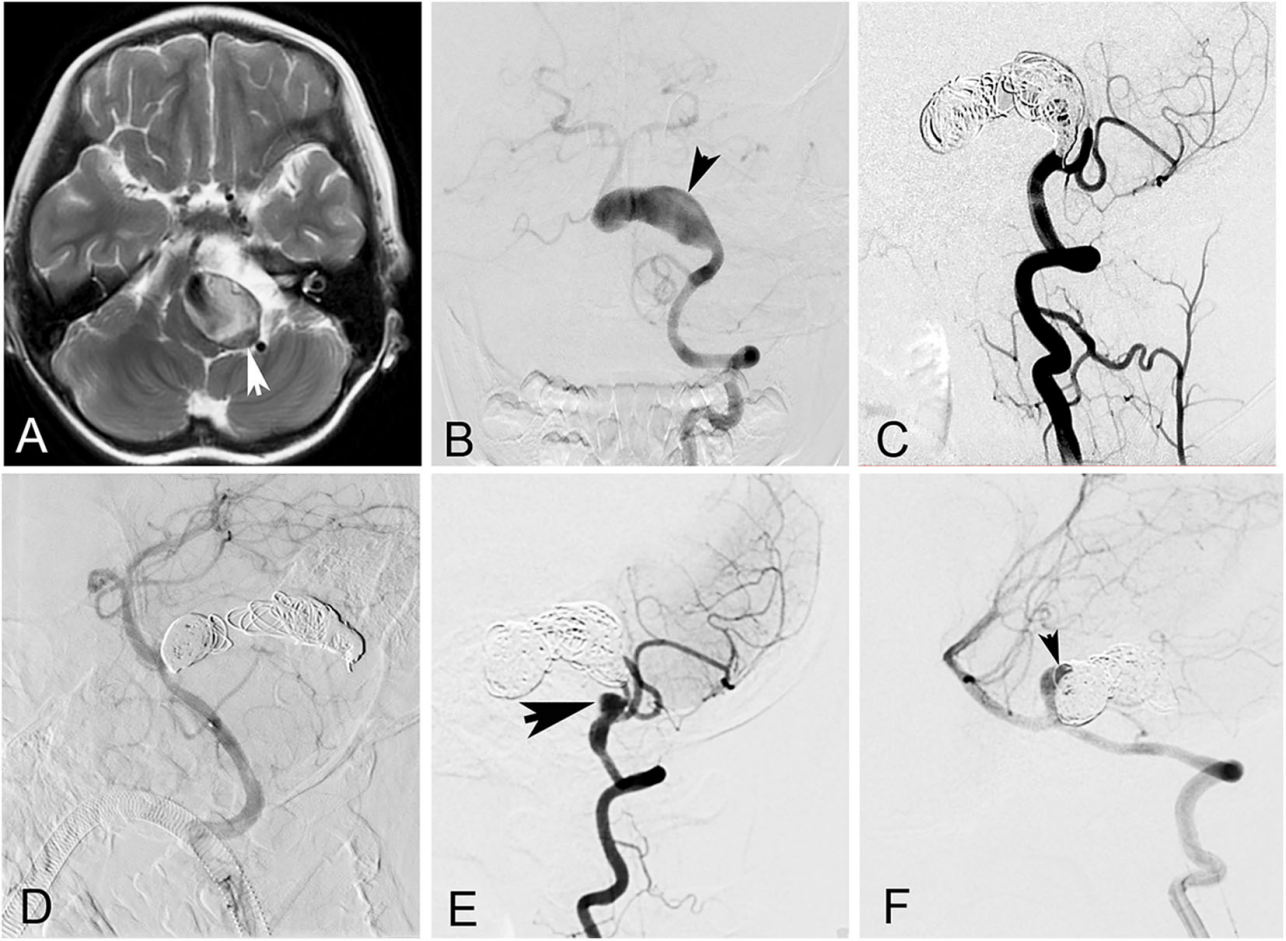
A 6-year-old girl with a giant vertebrobasilar dissecting aneurysm. (A) Magnetic resonance imaging showed a giant vertebrobasilar dissecting aneurysm with intramural hematoma. (B) Left vertebral anteroposterior angiogram revealed the aneurysm. (C) Left vertebral artery and the aneurysm were occluded with coils at lateral angiogram. (D) Right vertebral was patency after the procedure at lateral angiogram. (E,F) Left and right vertebral lateral angiogram at follow-up showed the aneurysm recanalized (arrows).
